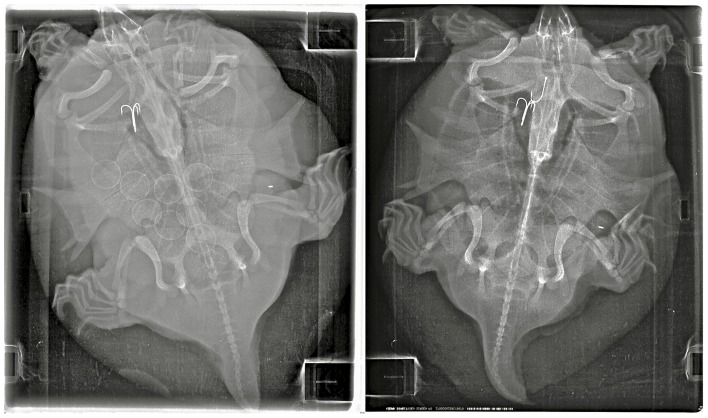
A Spiny Softshell (*Apalone spinifera*) first captured on 4 June 2012 (left) while gravid with eight eggs and containing one treble fish hook and again captured on 27 July 2012 (right) with an additional fish hook. A Passive Integrated Transponder (PIT tag) is visible in both X-rays. Image has been enhanced to improve hook visibility.
